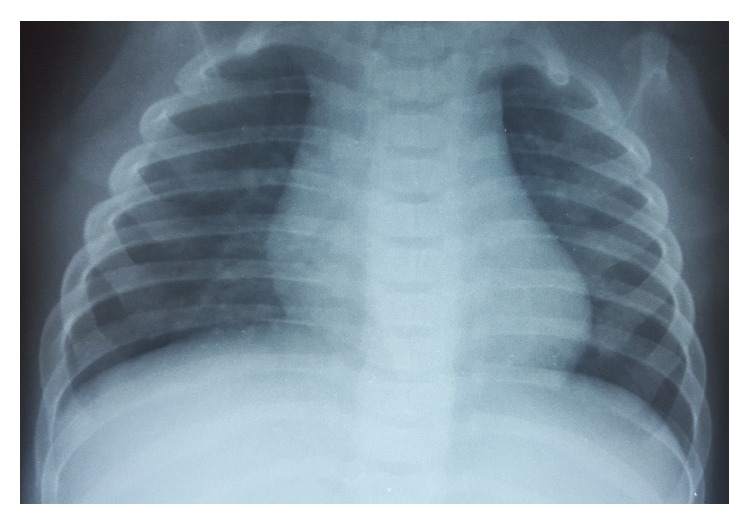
Normal chest X-ray on follow-up.
